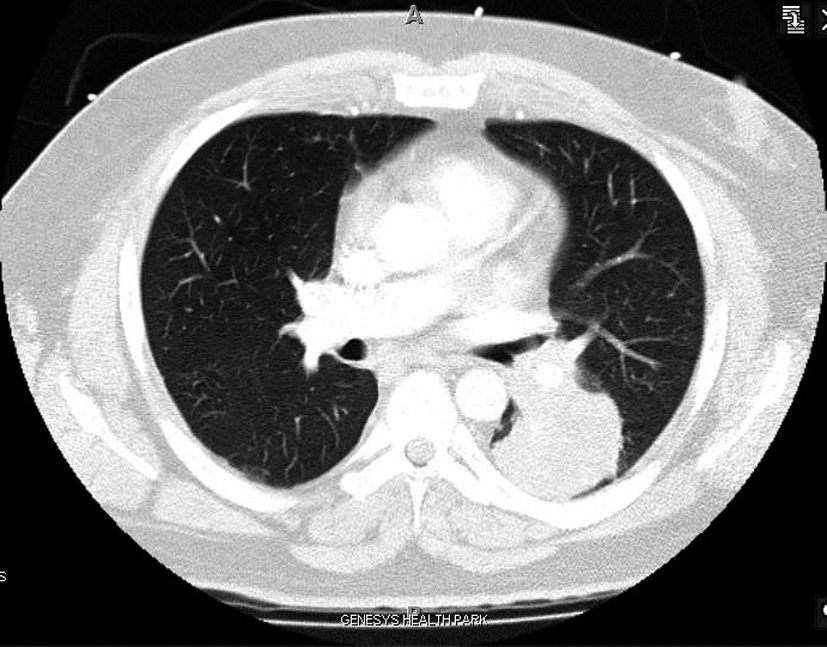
Sarcomatoid Carcinoma located in LLL on CT Angio.
